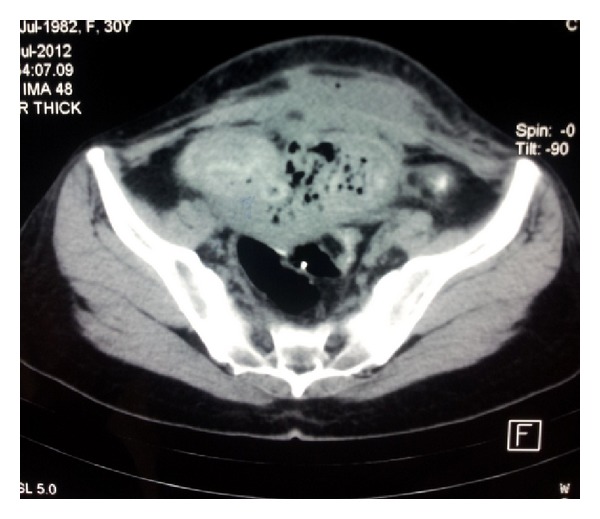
A CT scan showing retained surgical sponge next to the uterus.
